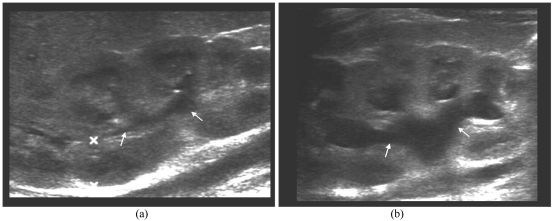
A one month-old child had history of antenatal bilateral hydronephrosis. a) Renal sonogram shows mild separation of right renal sinus (arrows); b) ECS shows increased dilatation of right renal pelvis (arrows) without presence of microbubbles. The MCU was normal.
